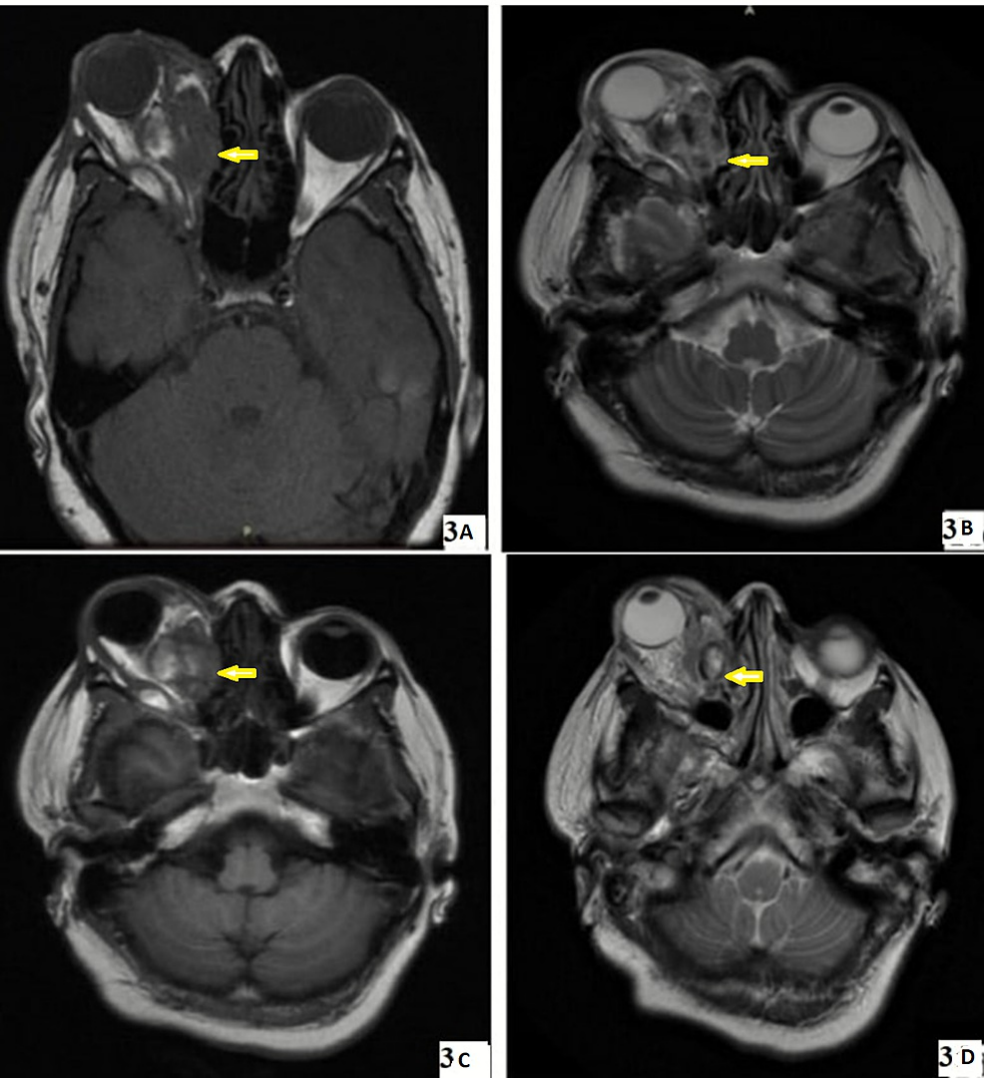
Contrast-enhanced magnetic resonance imaging of brain and orbit. (A) T1-weighted image. (B) T2-weighted image. (C) Diffusion-weighted imaging/apparent diffusion coefficient image. (D) Flair image. (A-D) Showing an ill-defined mass involving both intraconal and extraconal spaces without any evidence of intracranial extension (yellow arrows).
